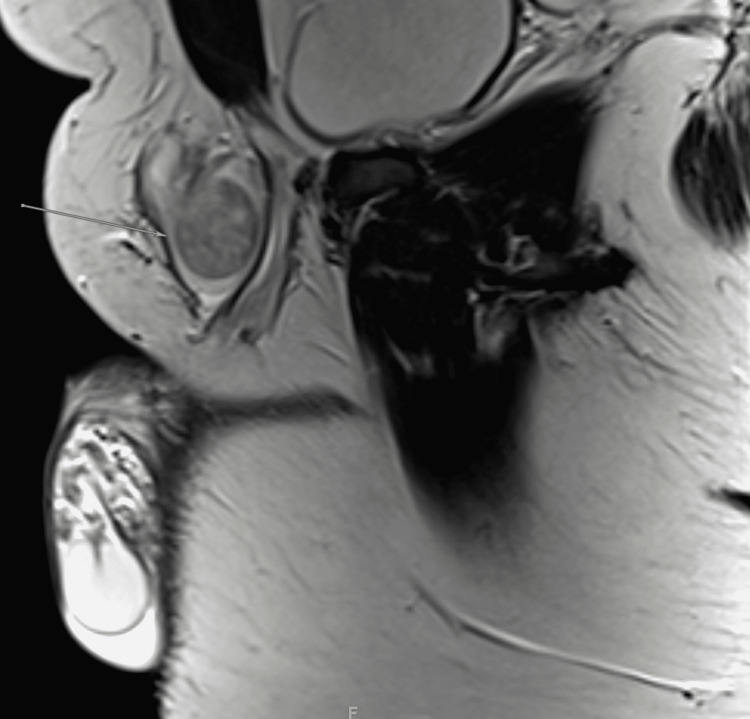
Sagittal T1-weighted MRI. Well-defined left inguinal canal lesion abutting the anterior aspect of the spermatic cord. The mass measured 3 x 3 x 5.5 cm in maximum anteroposterior, transverse, and craniocaudal diameter, respectively. The lesion demonstrates low intensity
